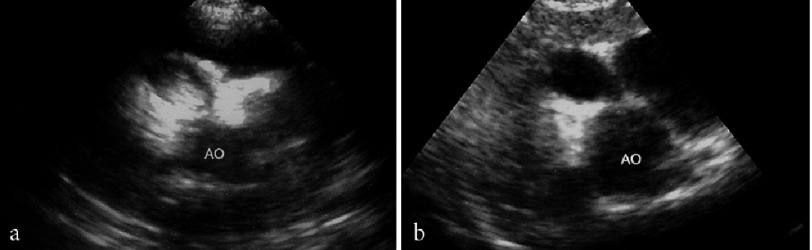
Blood clot regression in giant aneurysm of right coronary artery. a - aneurysm of proximal segment of right coronary artery with a diameter of 10 mm and large thrombus was revealed using echocardiography first performed in 2 months after KS onset, b – in 3 months thrombus in the aneurysm is not visualized.
